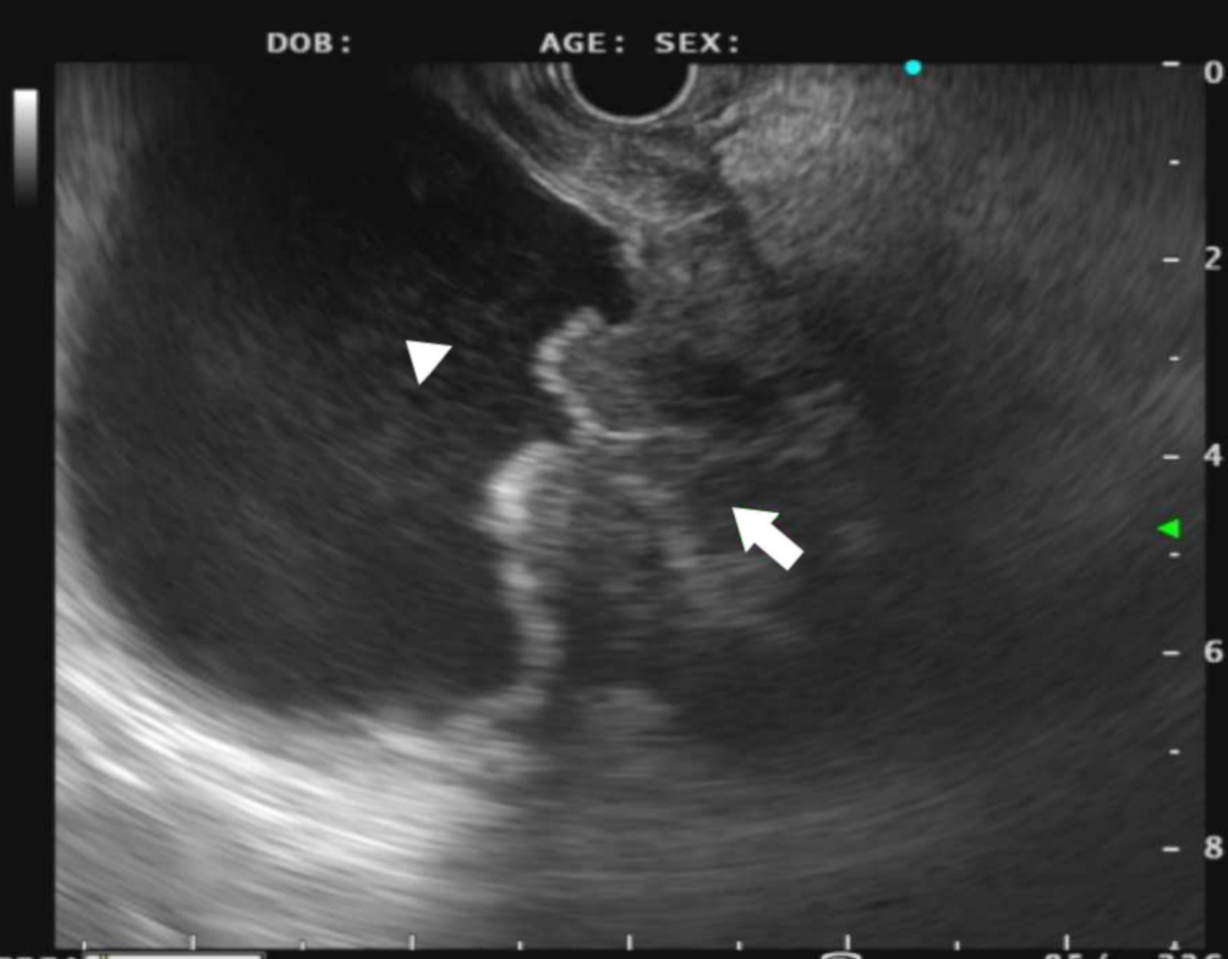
Endoscopic ultrasound (EUS) showing antral wall thickening in the excluded stomach (solid arrow)
